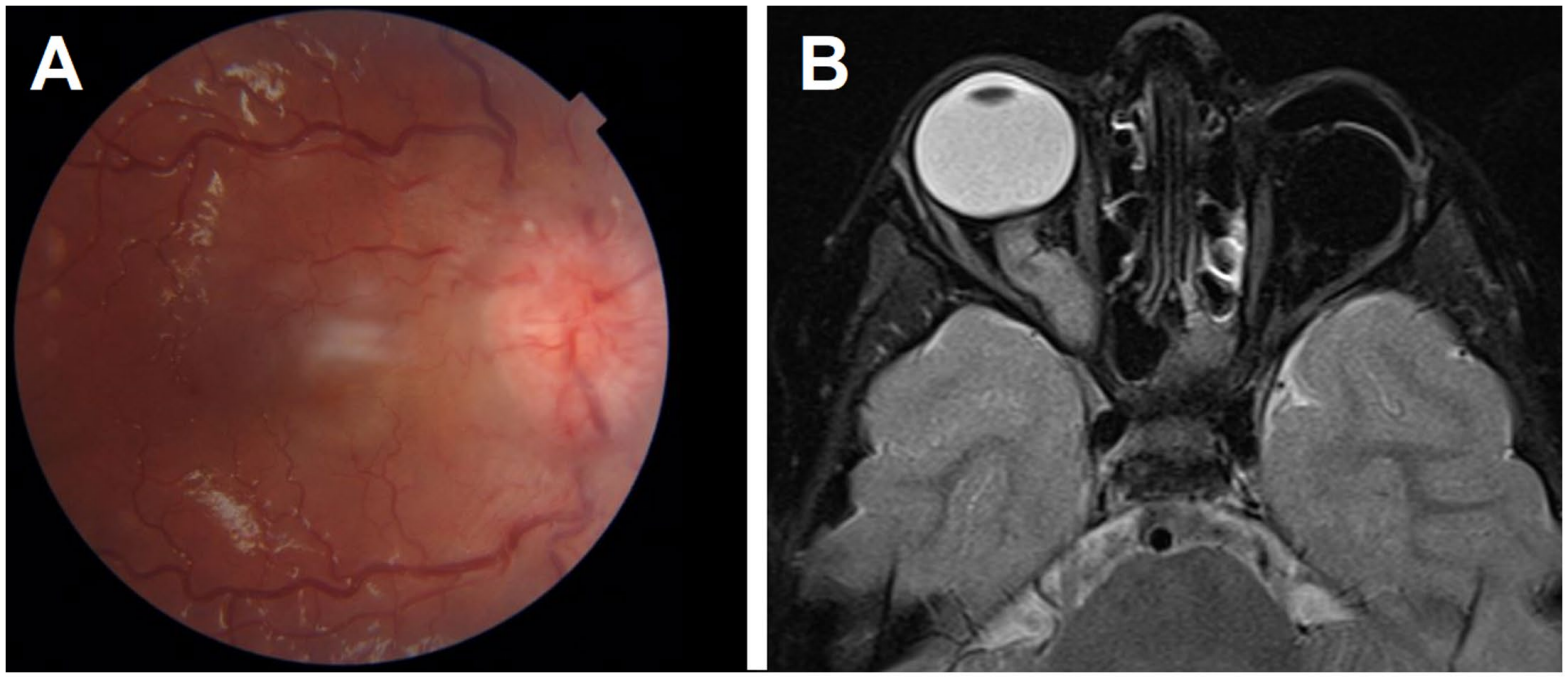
A patient with left unilateral retinoblastoma had her eye enucleated and presented later with right optic disc edema (A). T2 MRI showed thickening and contrast enhancement of the right intra-orbital optic nerve indicating metastatic Retinoblastoma. This was associated with leptomeningeal metastasis (B).
